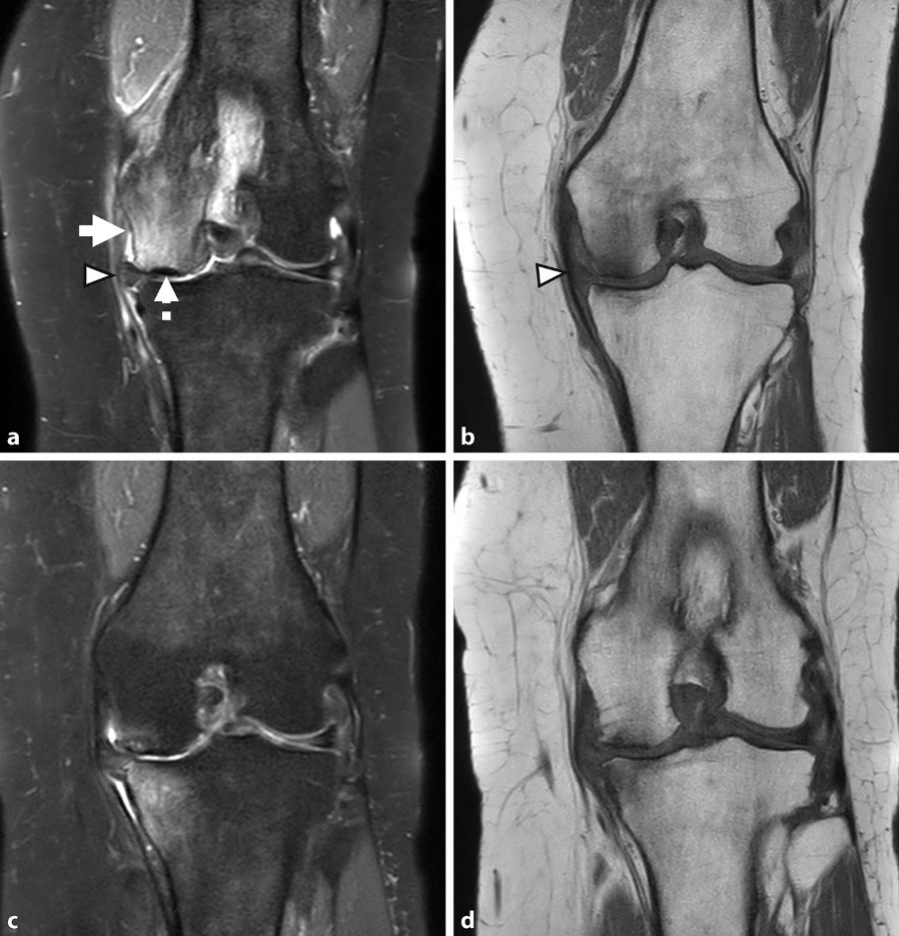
Irreversible, high grade subchondral insufficiency fracture of the knee of the medial femoral condyle (MFC). Coronal Proton Density with fat suppression (a) and T1-weighted imaging (b): there is diffuse bone marrow edema in the MFC (*arrow*). In addition, there is a band of low signal intensity compatible with osteonecrosis of the subchondral fragment (*dotted arrow* in a). Note the severe medial meniscal body extrusion (*arrowhead*). The contour of the articular surface is maintained. There is diffuse cartilage loss. Coronal proton density with fat suppression (c) and T1-weighted imaging (d) after 1 year. There is advanced osteoarthritis with complete cartilage loss, joint space narrowing and osteophytes
